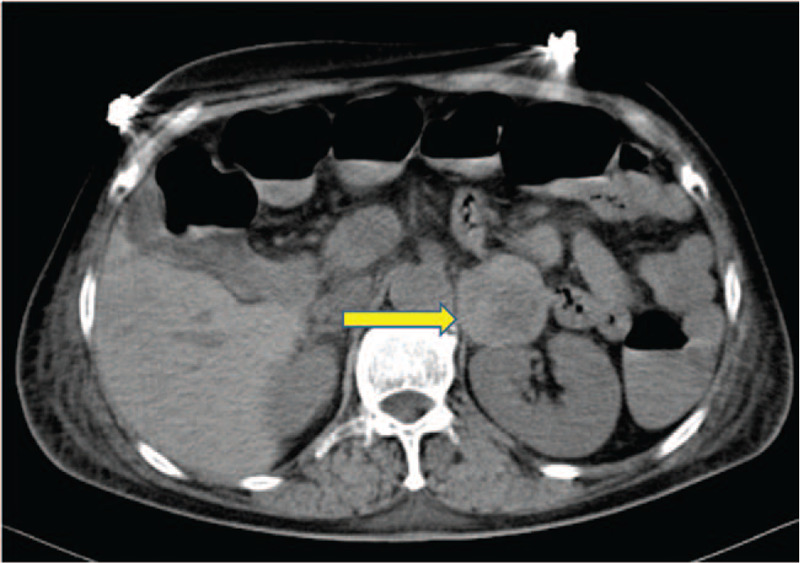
Computed tomographic scan demonstrating a left adrenal mass (arrow).
